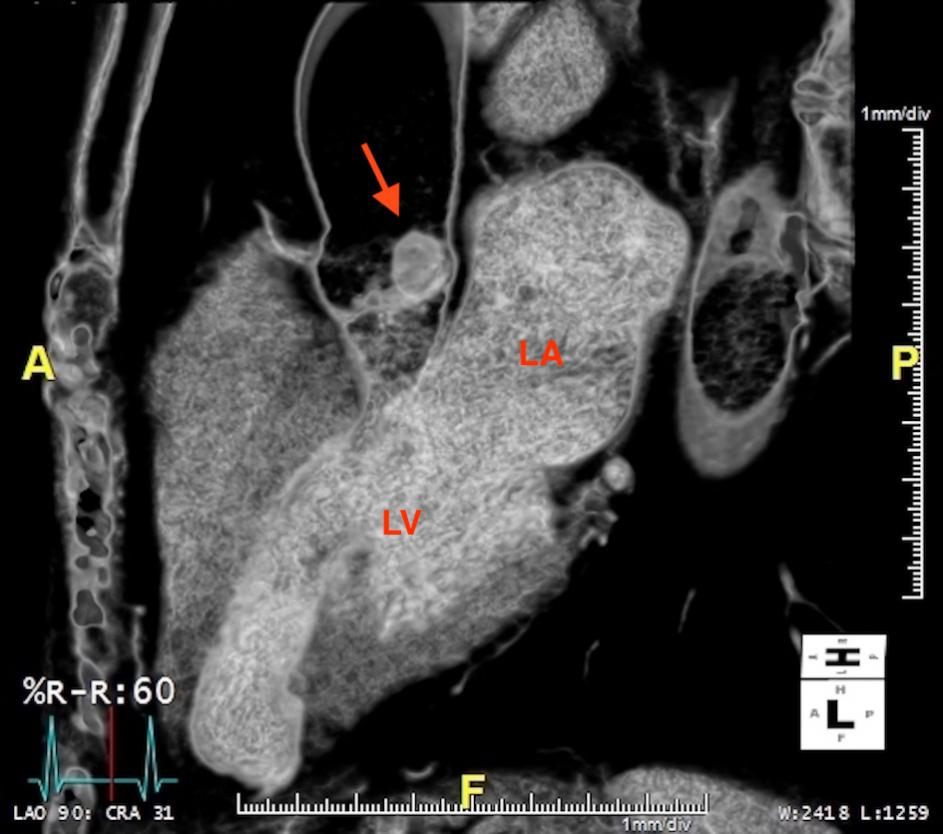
3-D CT of the chest in diastolic phase .
